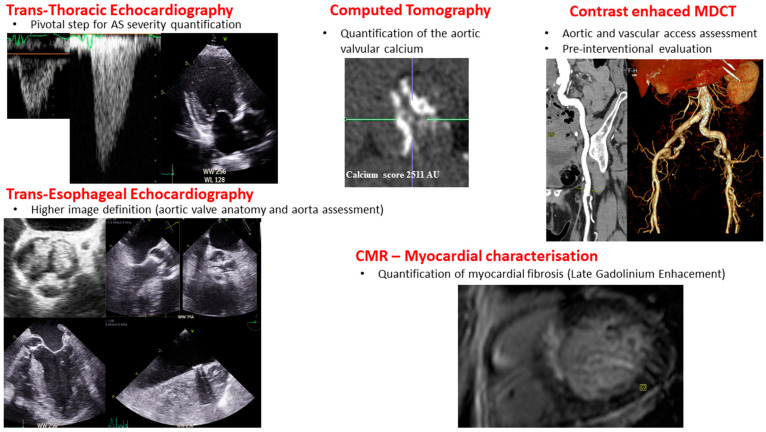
Multi-imaging modalities in aortic stenosis diagnosis. Legend: AS: aortic stenosis, CMR: Cardiac Magnetic Resonance, MDCT: Multi-Detector Computed Tomography.
